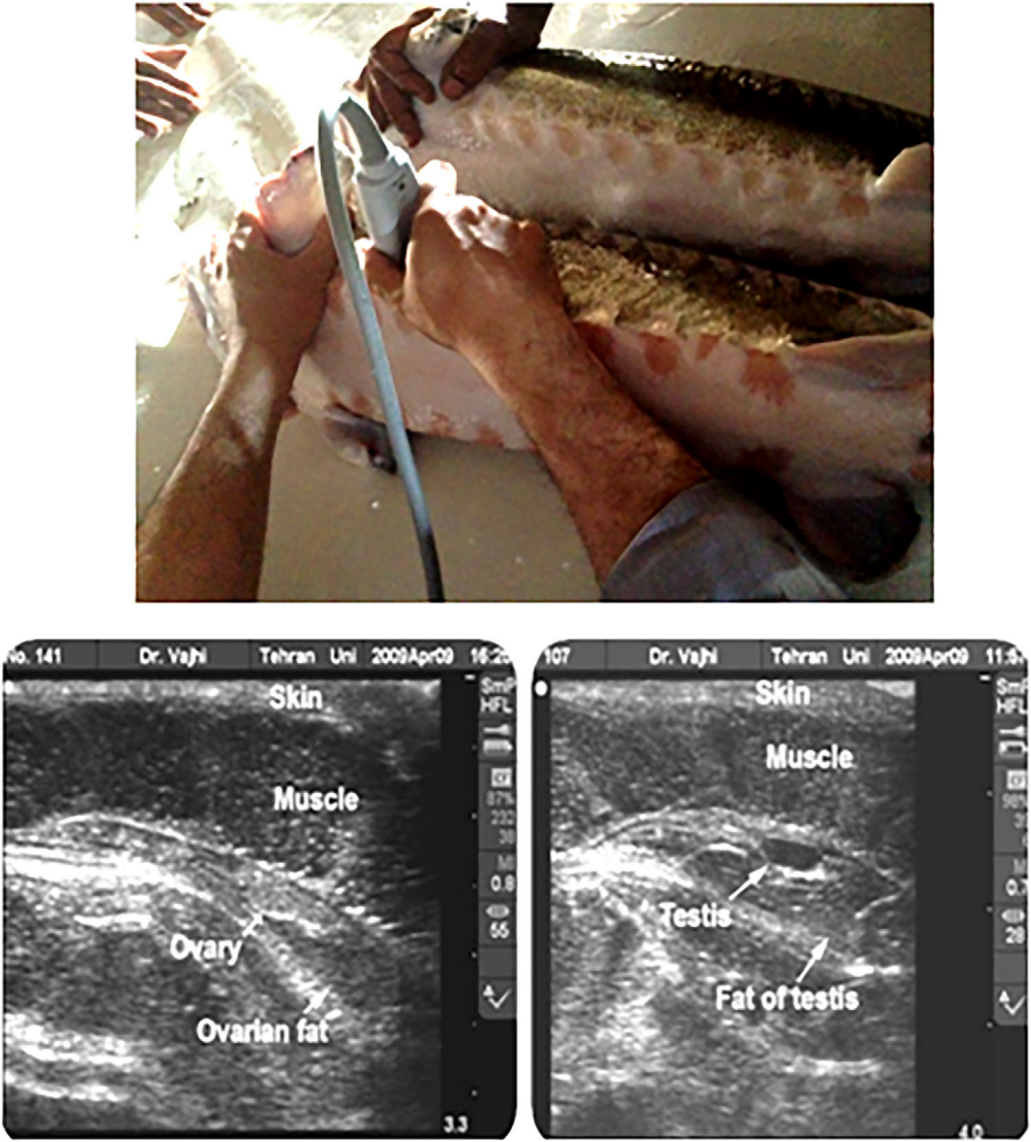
Determination of the gender in each broodstock sturgeon farm based on ultrasound method.
